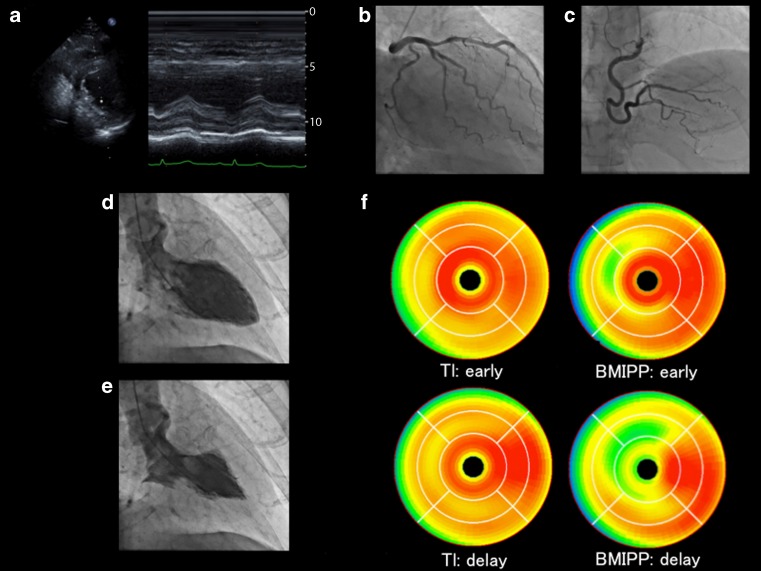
a Transthoracic echocardiogram on arrival showing akinesia of the anteroseptal wall, b and c Emergency coronary angiograms. Neither the left coronary artery nor the right coronary had occlusion or significant stenosis. d and e Left ventriculograms. The mid-left ventricle was severely hypokinetic. f Single photon-emission computed tomogram, polar and heart score view maps of thallium myocardial perfusion and BMIPP images. *BMIPP* iodine-123-labeled 15-(p-iodophenyl)-3-R′S‐methylpentadecanoic acid, *TI* thallium 201
